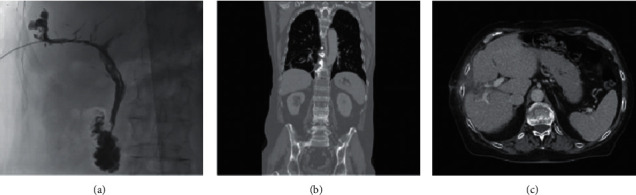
Percutaneous cholangiography (a) and chest-abdomen CT scan (b, c) showing resolution of previous bile leakage.
